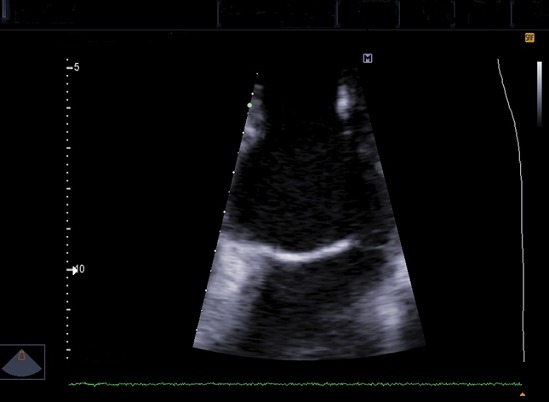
Échocardiographie doppler cardiaque de contrôle réalisée après 4 semaines de traitement montrant la disparition de végétation au niveau petite valve mitrale
